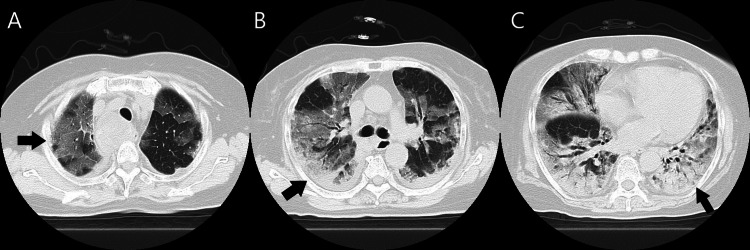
(A-C) Chest computed tomography images on day 1 Multiple bilateral ground-glass opacities are evident in all lobes, and consolidation is evident in both lower lobes of the lungs.
